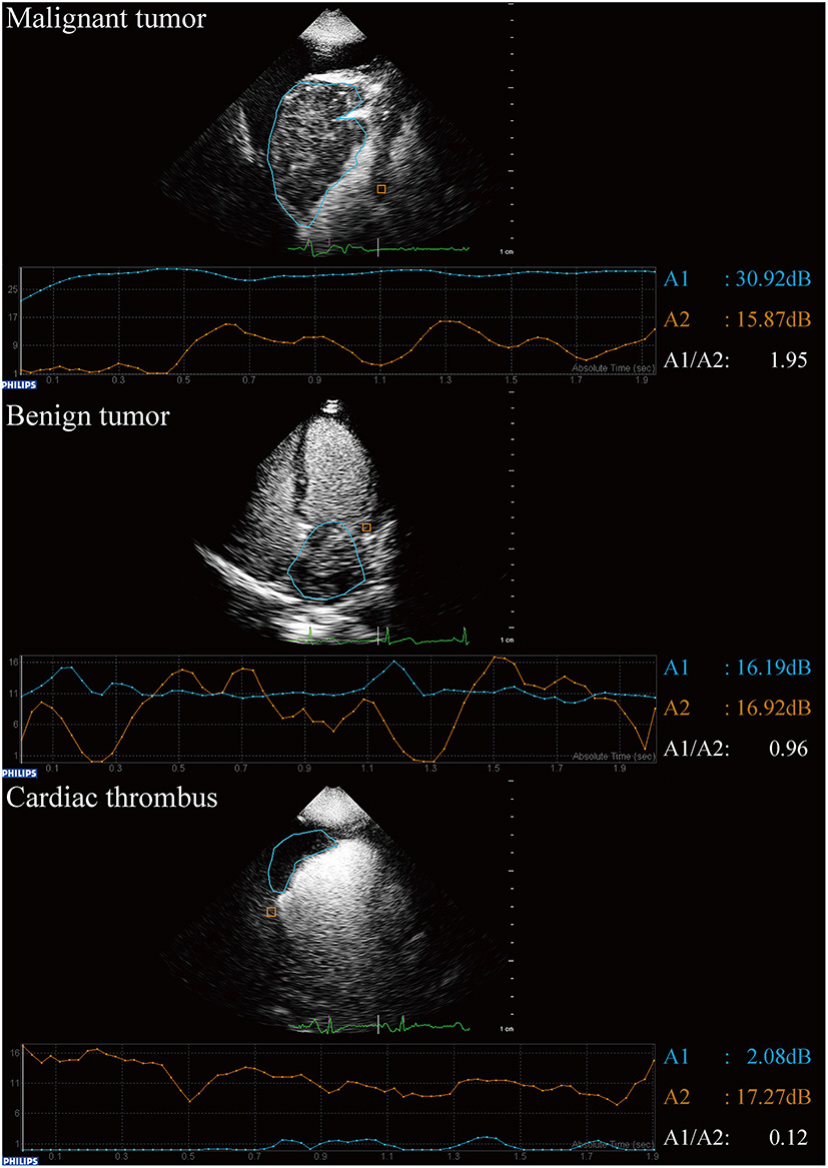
Qualitative analysis of contrast echocardiography in patients with suspected cardiac masses using QLAB software.
